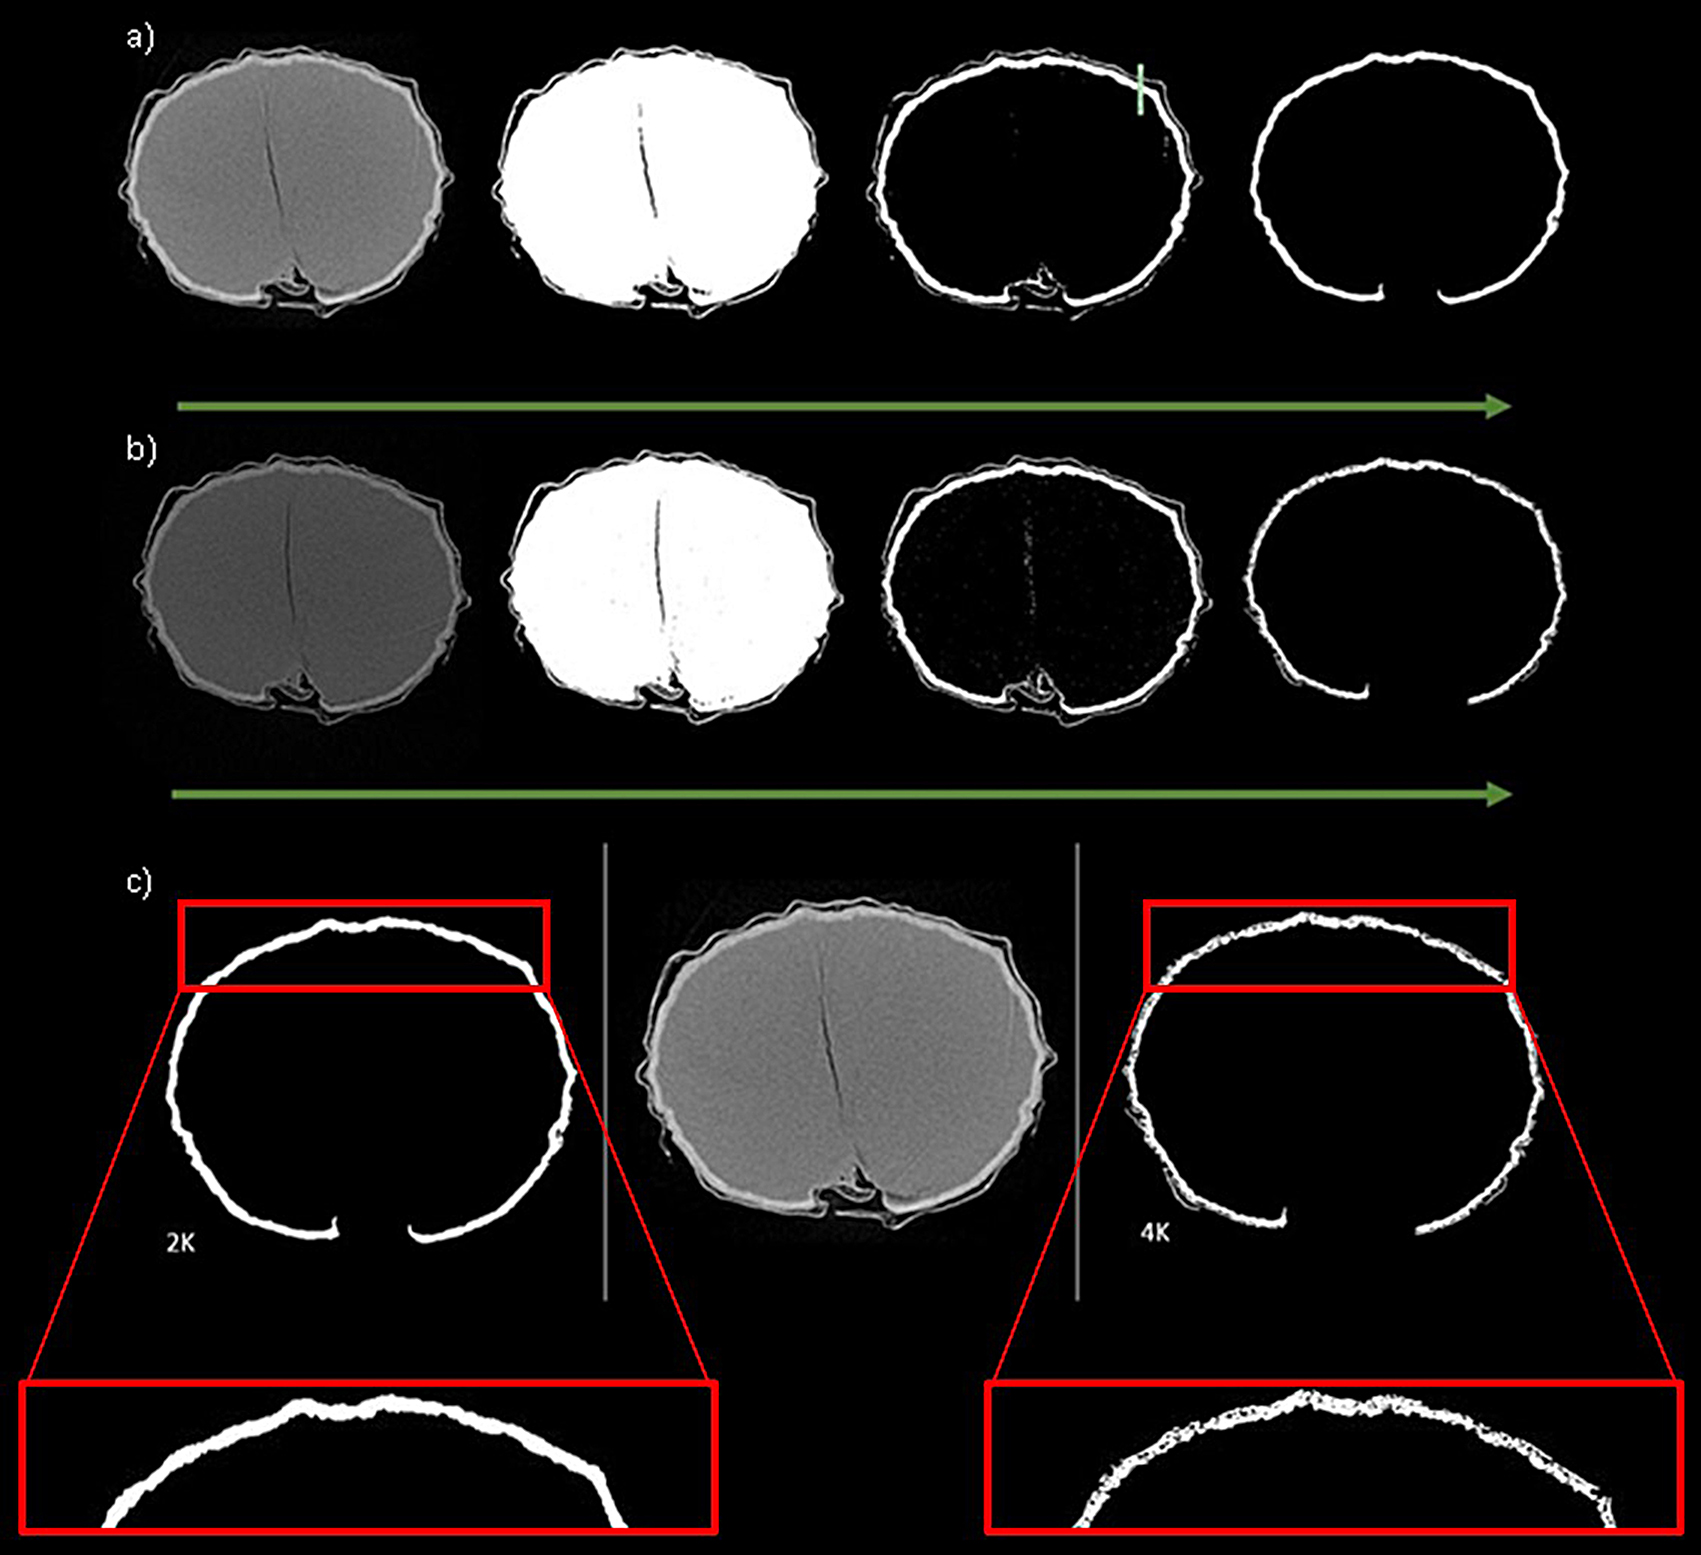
Thresholding in CTAn of grains scanned using 2K and 4K camera binning to identify most appropriate time vs. image quality and resolution. (a) using the 2K camera binning, images left to right, starting with x-ray image of a grain cross section, binarized image isolating the whole grain, binarized image isolating the aleurone layer by density, post despeckling function, removing husk/leaving the largest object which is the aleurone layer. (b) using 4 K camera binning, left to right, starting with x-ray image of a grain cross section, binarized image isolating the whole grain, binarized image isolating the aleurone layer by density, post despeckling function, removing husk/leaving the largest object which is the aleurone layer. (c) side by side comparison of the isolated aleurone layer comparing the result when using 2K camera binning (left), 4K camera binning (right) and an Xray image (center) for comparison
